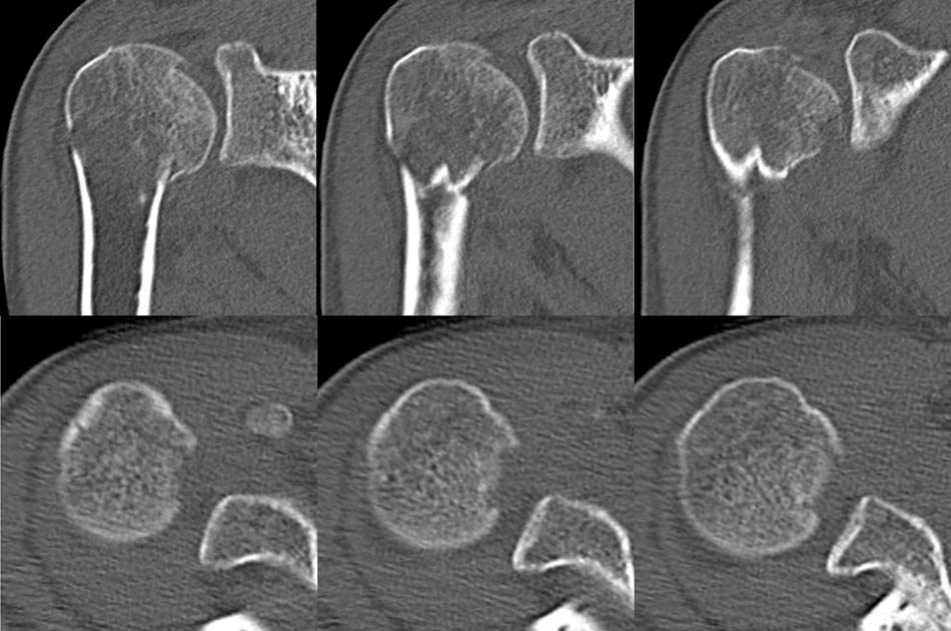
Original CT scan showing impression fracture and surgical neck fracture.
